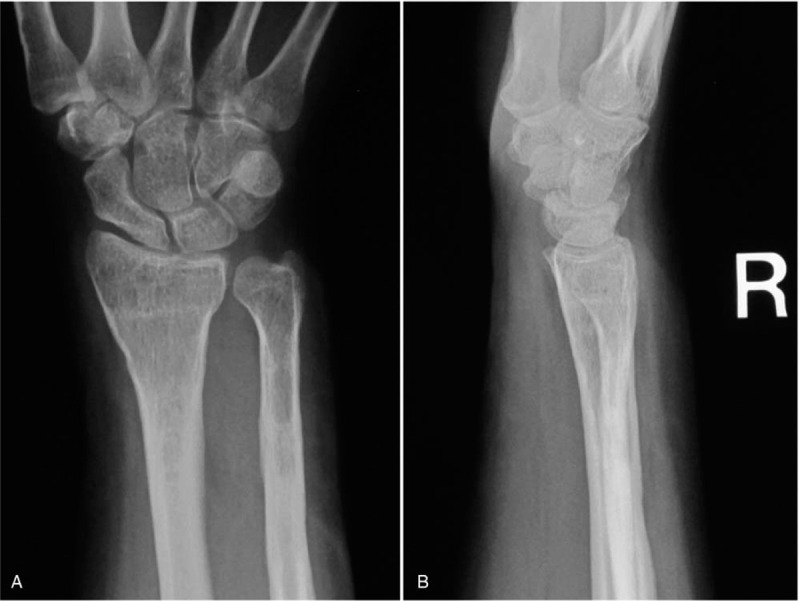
Presenting anteroposterior (A) and lateral (B) radiographs of her right wrist at a year and 9 months after the operation. The radiocarpal and distal radioulnar joint was restored.
